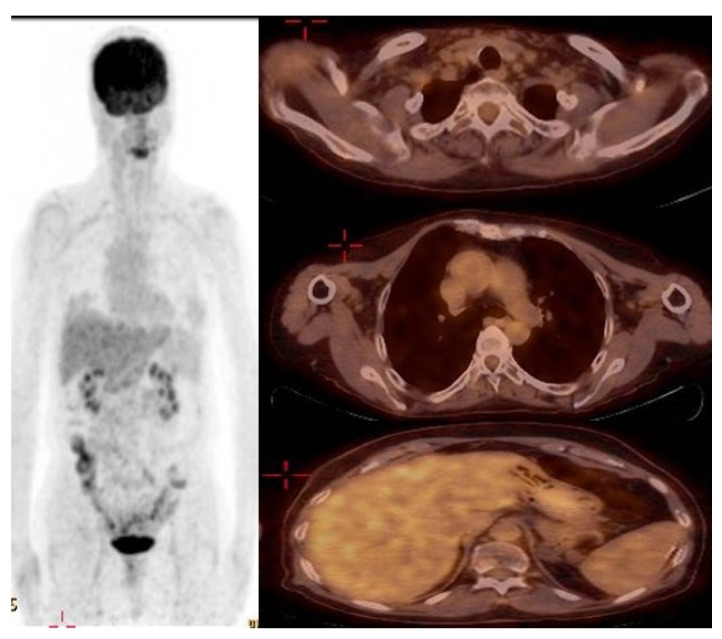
PET-CT showing complete radiologic response to Osimertinib treatment.
